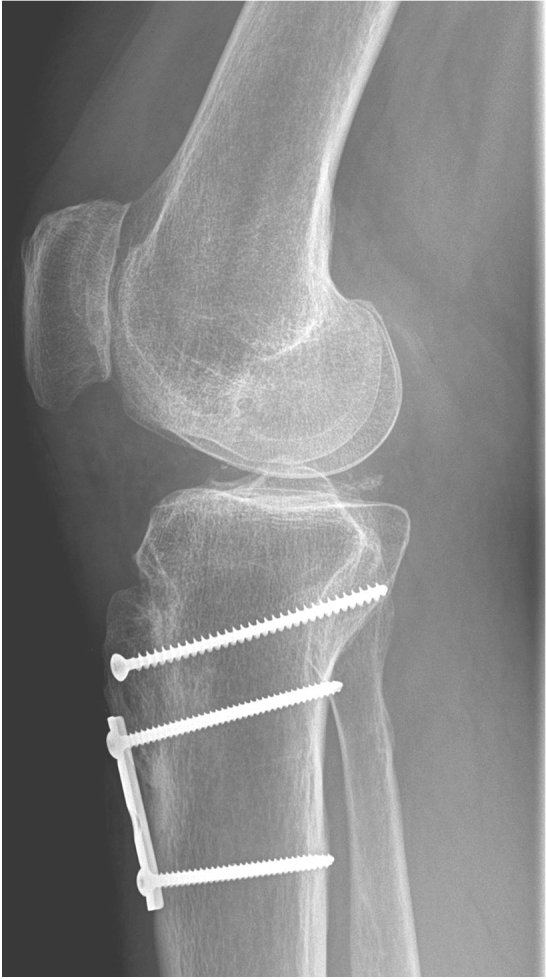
Example lateral X-Ray of a standard fixation of the tibial tuberosity fragment using a one-third-tubular plate, proximal lag screw and two distal cortical screws
